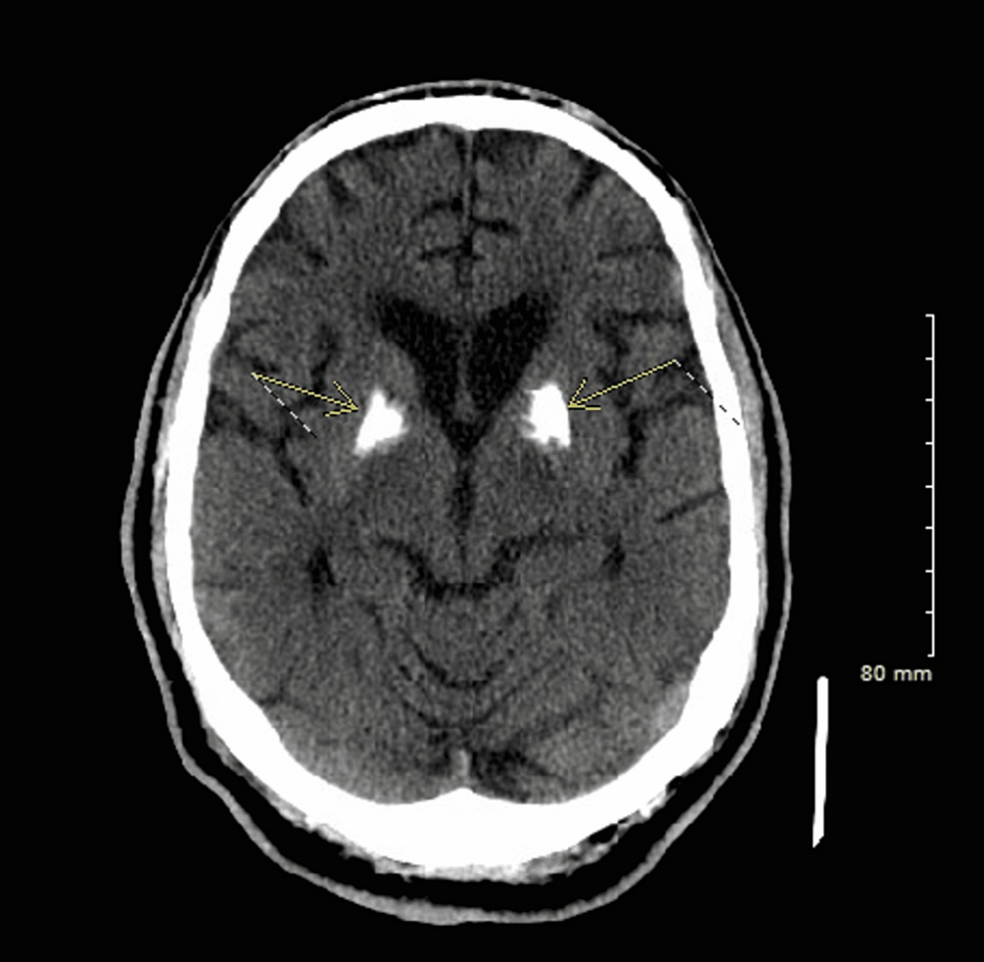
CT of the head shows mild, vague patchy areas of low attenuation in the periventricular white matter, likely ischemic/hypertensive in nature Dense bilateral near-symmetric areas of increased density/mineralization in the left and right basal ganglia and to a lesser degree, the left and right posterior thalamus dentate nuclei are seen as well, likely representing Fahr’s disease.
